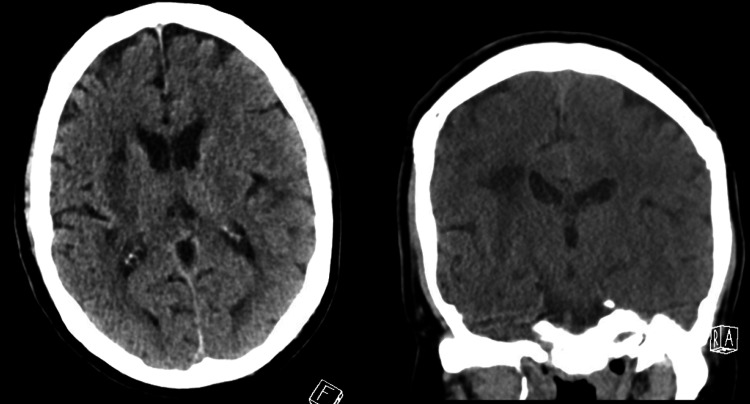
Post-operative CT scan for patient 3 Post-operative CT scan with complete evacuation of sICH sICH: Spontaneous intracerebral hemorrhage
